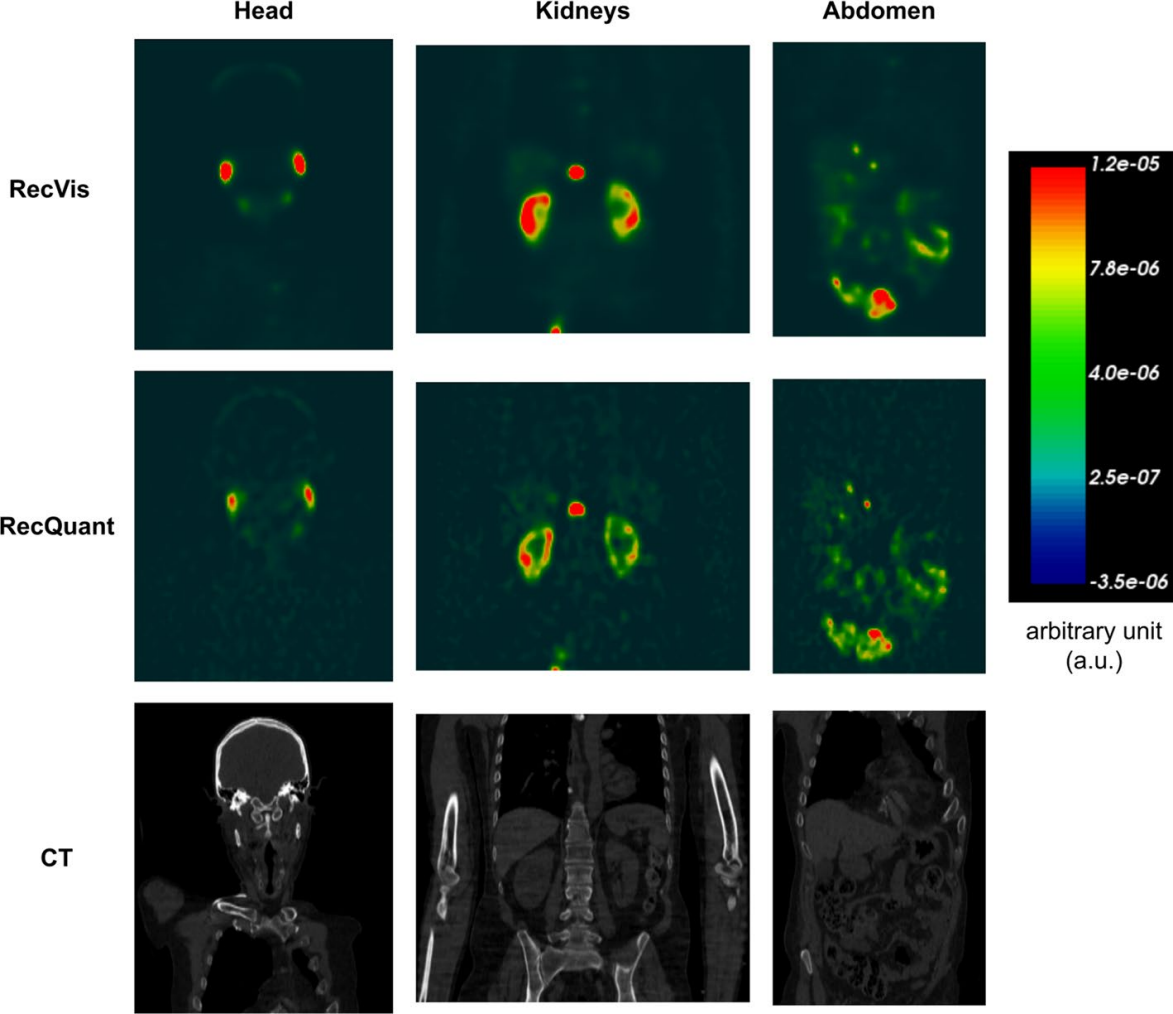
Normalised SPECT reconstructions (RecVis and RecQuant) in arbitrary unit of a patient treated with ^177^Lu-PSMA-1 24H after the first injection. The focus is on three regions: the head, the kidneys and the abdomen. Associated CT scans are also available
